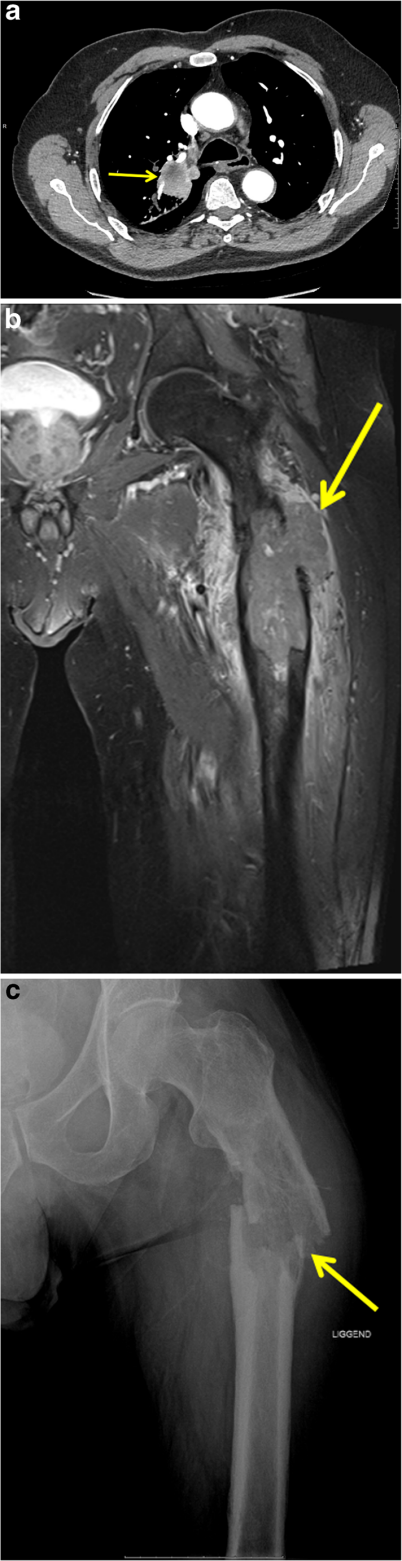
Pathological femoral shaft fracture in a 63-year-old man with a stage IV bronchogenic carcinoma. a Axial chest CT image in mediastinal window setting shows a large tumour in the right upper lobe (*yellow arrow*). b Coronal FS T1-weighted MR image after gadolinium contrast administration depicts a mass in the proximal left femoral shaft with extra-osseous soft tissue component (*yellow arrow*) and significant surrounding soft-tissue oedema. The patient presented to the emergency department 1 week after the MRI with complaints of extreme pain (non-traumatic) in the left hip and immobility. c Supine X-ray of the left femur reveals a pathological fracture with angulation at the level of the lytic bone metastasis (*yellow arrow*)
